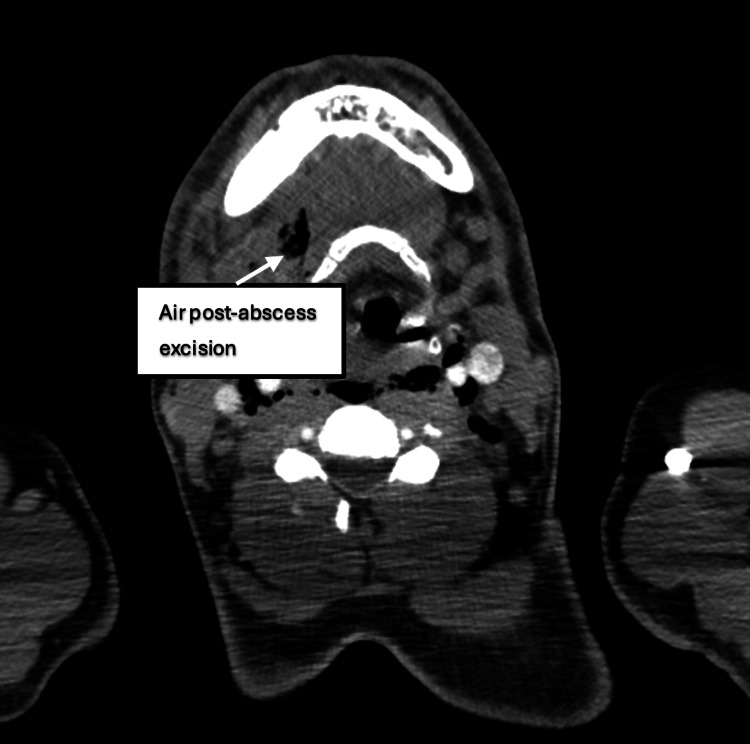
CT of the neck showing the presence of air following the excision of abscess (day 5). CT: computed tomography
